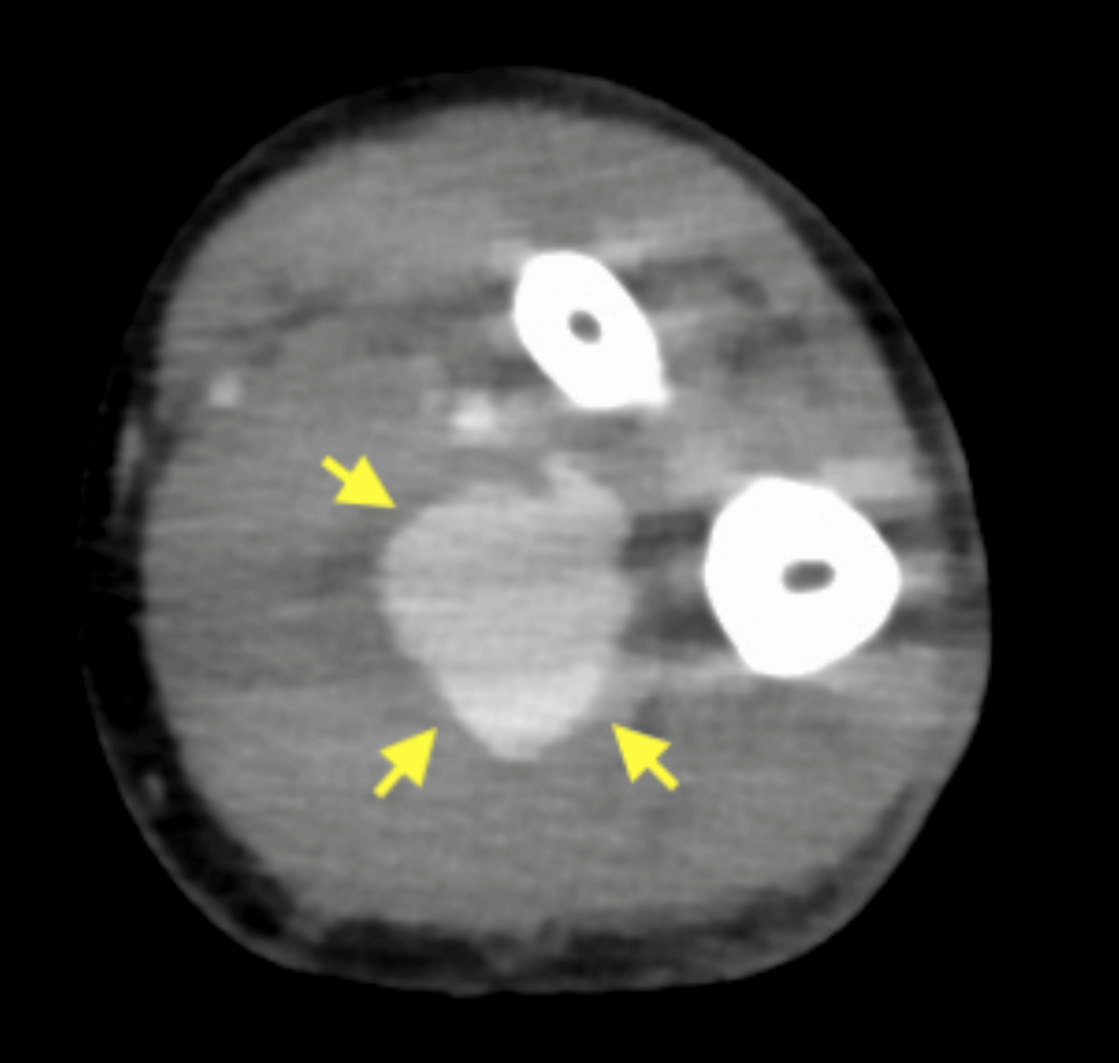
Contrast-enhanced computed tomography of the left forearm Contrast-enhanced computed tomography (CT) showing extravasation (yellow arrow) from the ulnar artery.
